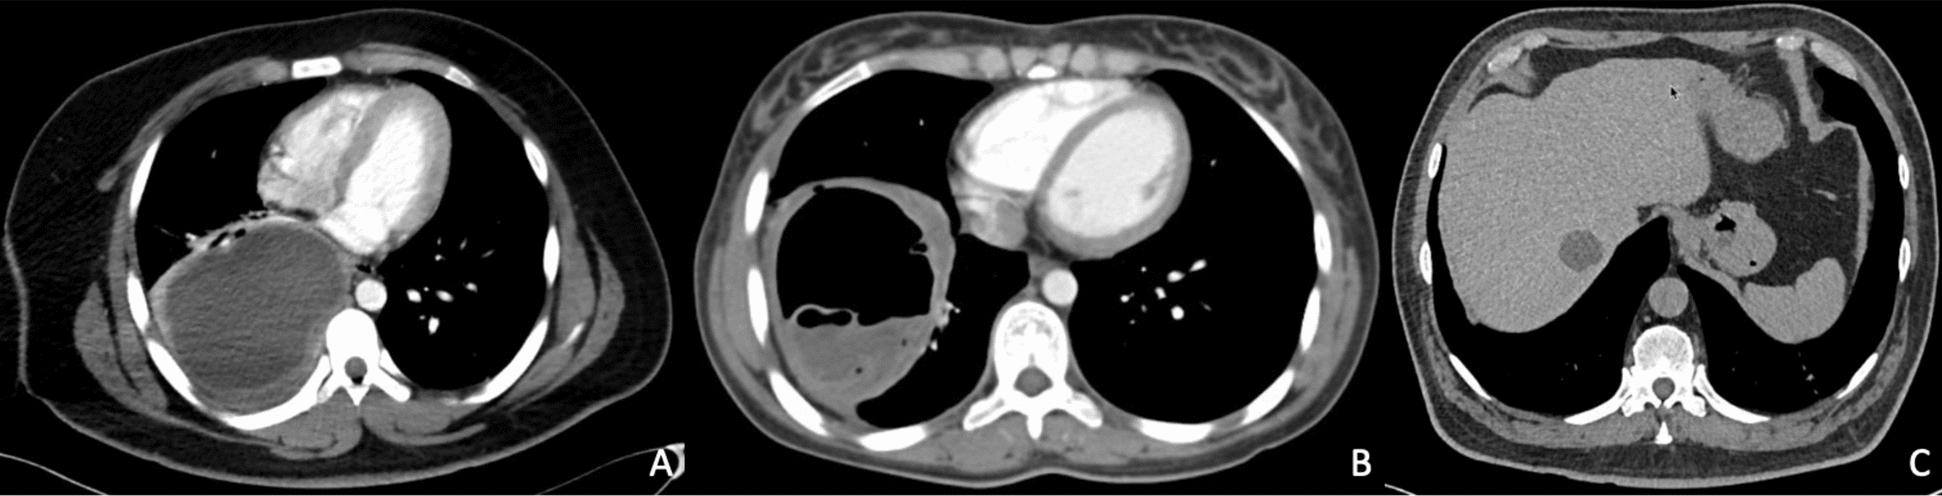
Cross-sectional contrast-enhanced CT scans of different cases. A Ruptured cyst is contained in the right lower pulmonary lobe with the characteristic crescent sign and the water lily sign (floating membranes due to the collapse of the endocyst). B Giant lung cyst (> 10 cm) uncomplicated. C Shows a single uncomplicated hepatic cyst in hepatic region VI
